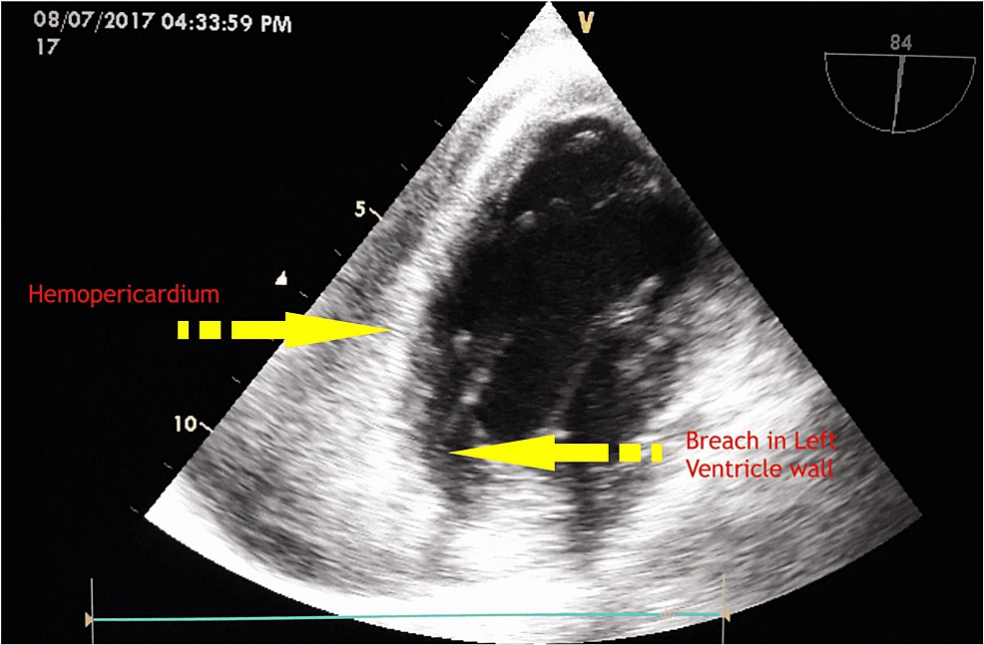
Echocardiographic image showing a breach in the continuity of the inferolateral myocardial wall
